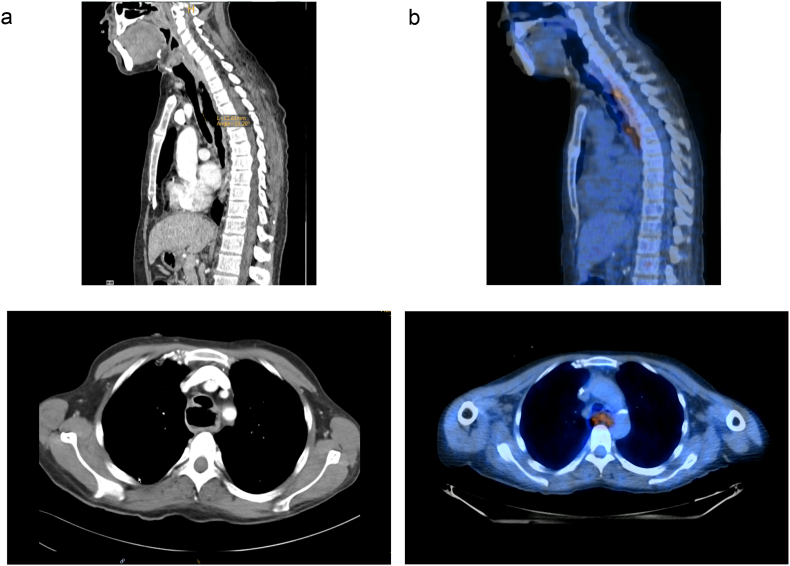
Initial imaging. a) CT-scan sagittal (top) transversal (bottom) showing extensively dilated and wall-thickened esophagus (42 × 23 mm), a tracheo-esophageal fistula (6 × 13 mm) on the level of the T3 vertebral body. b) PET CT scan sagittal (top) transversal (bottom) showing a slightly activated 11.5 cm long esophagus (middle third, SUV max 7.4) without locoregional lymphadenopathy.
